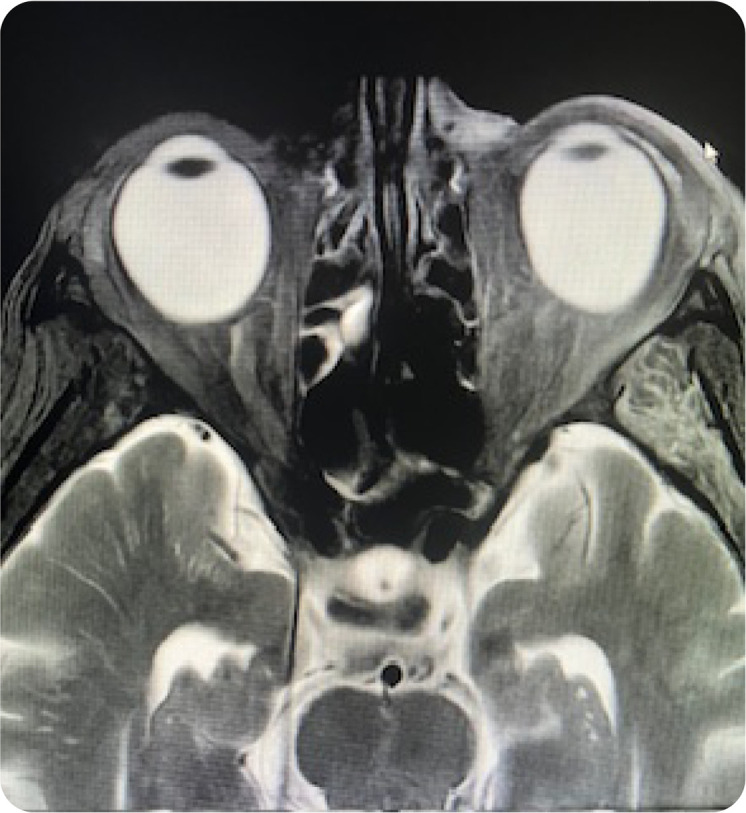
Orbital MRI showing increased signal intensity in the subcutaneous tissues of the left orbital preseptal area, partial retroorbital involvement, and mild proptosis.
